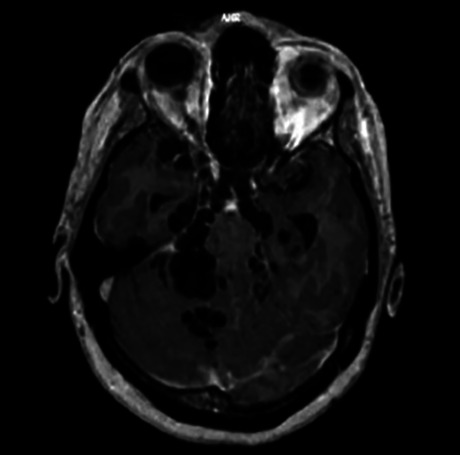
MRI subarachnoid racemose cysticercosis, involving basal cisterns.
